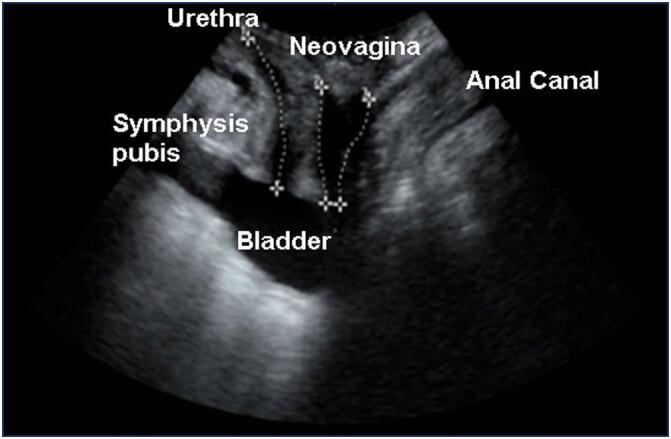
Transperineal ultrasound image in the mid-sagittal plane during Valsalva maneuver in transgender women submitted to gender-affirming surgery
